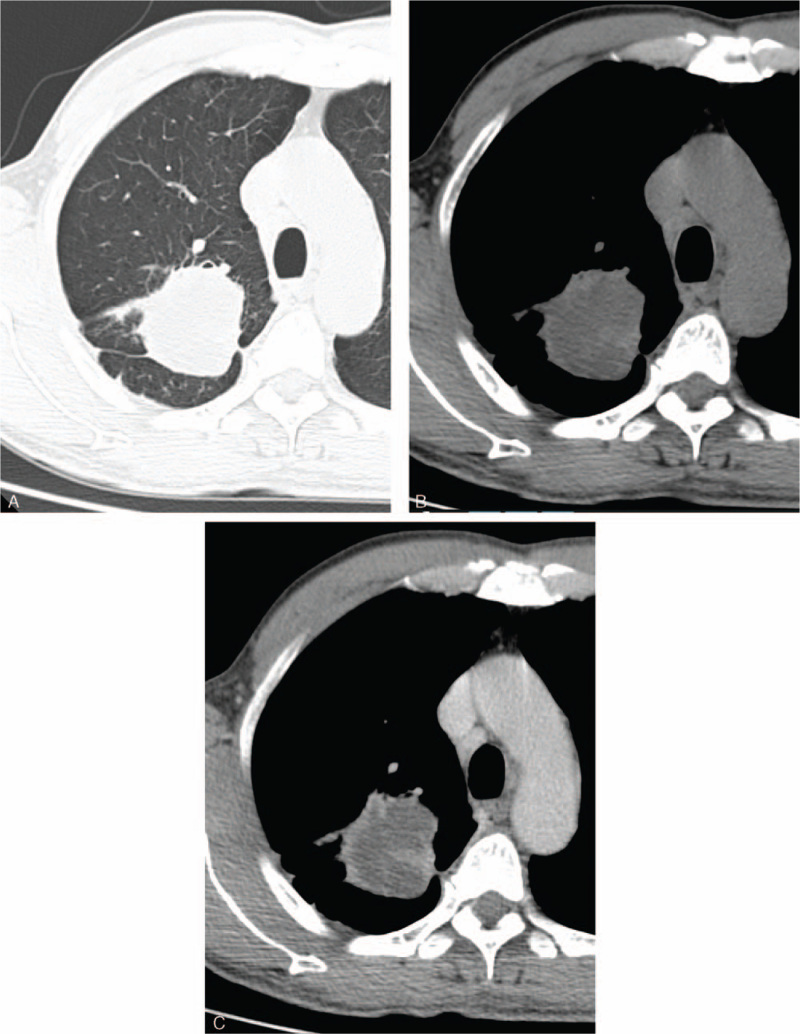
Chest contrast-enhanced CT revealed an analogous round mass in the posterior segment of the right upper lobe with heterogeneous enhancement, and extensive central liquefaction, a few striped shadows around the tumor. (A) CT plain scan of lung window; (B) CT plain scan of the mediastinal window; and (C) Contrast-enhanced CT scan of the mediastinal window. CT = computed tomography.
